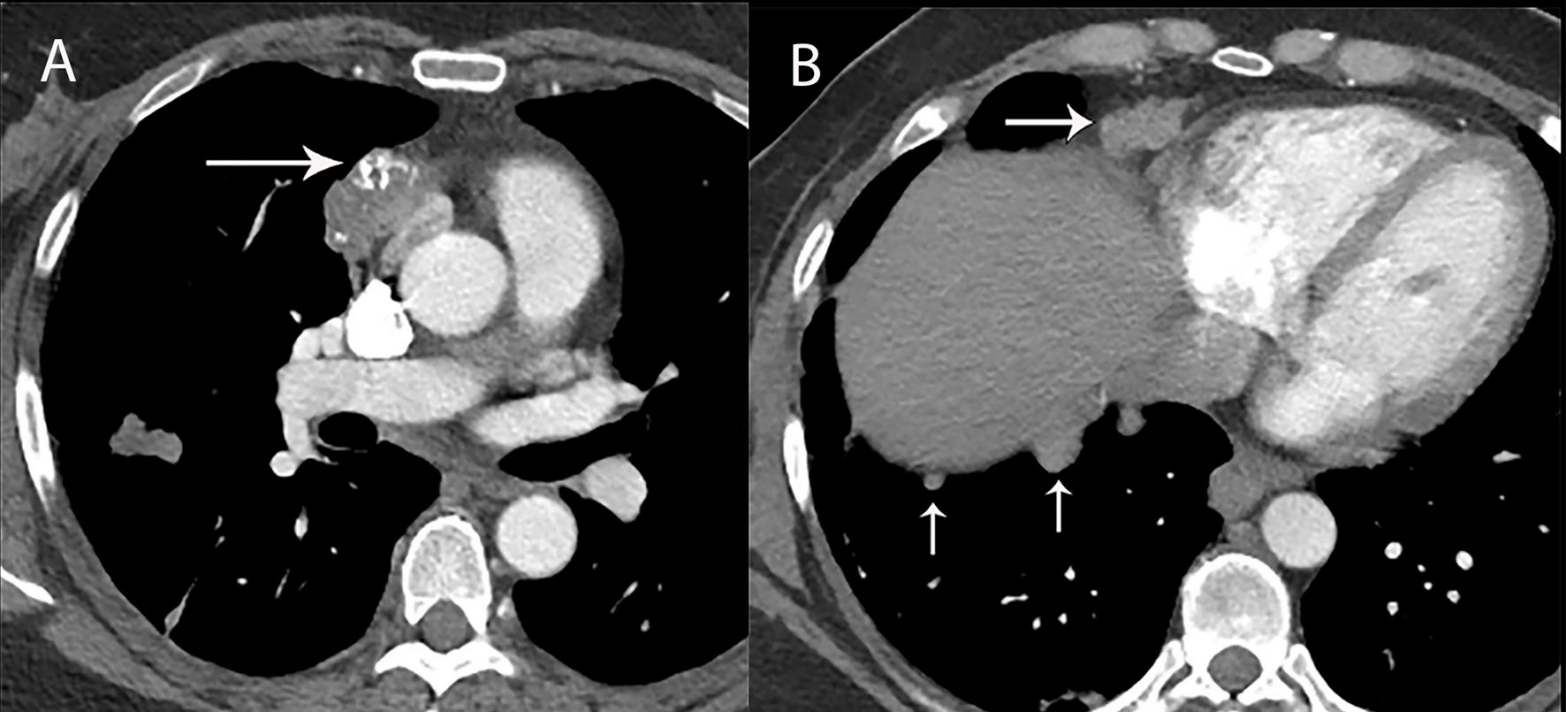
46 year old woman with thymic carcinoma with pleural metastases. (A) Contrast-enhanced CT shows right prevascular mediastinal mass (arrow) with heterogeneous attenuation and calcifications. (B) CT shows nodular right diaphragmatic pleural metastases (vertical arrows) and right anterior diaphragmatic nodal metastasis (horizontal arrow).
